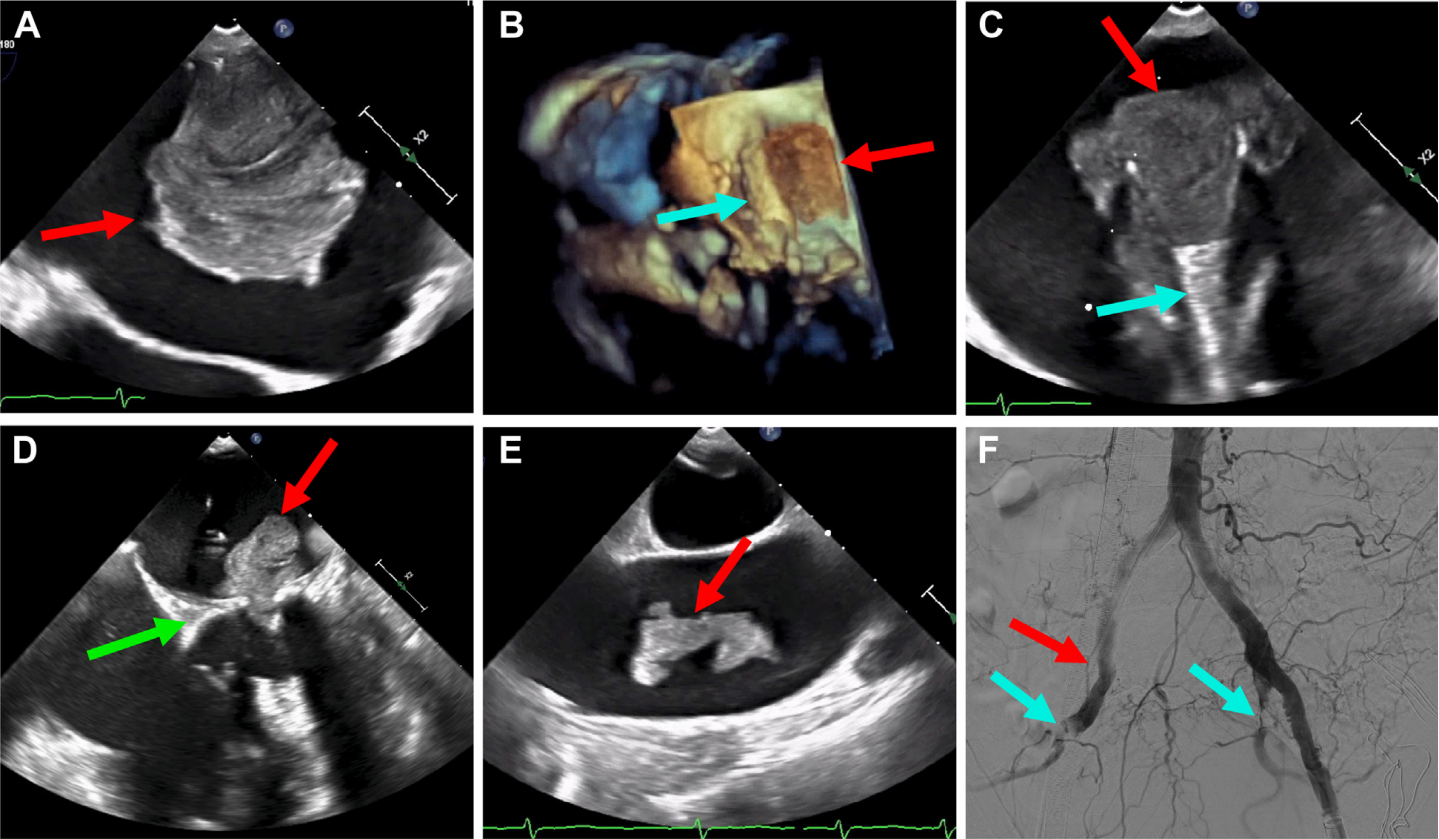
Transcatheter Aspiration of a Large Left Atrial Mass With an Embolized Fragment Occluding Peripheral Vasculature (A) Large left atrial (LA) mass (red arrow) as seen by transesophageal echocardiography (TEE). (B) Three-dimensional TEE showing large LA mass (red arrow). The aspiration catheter has been inserted into the left atrium (blue arrow) just adjacent to the LA mass. (C) The bulk of the LA mass (red arrow) being suctioned into the aspiration catheter (blue arrow). (D) A fragment of the LA mass (red arrow) broke off and is seen traversing the mitral valve (green arrow). (E) Embolized fragment of the LA mass (red arrow) in transit in the aortic arch. (F) Complete thrombotic occlusion of the ostial right external iliac artery (red arrow) and subtotal thrombotic occlusion of the proximal bilateral internal iliac arteries (blue arrows).
